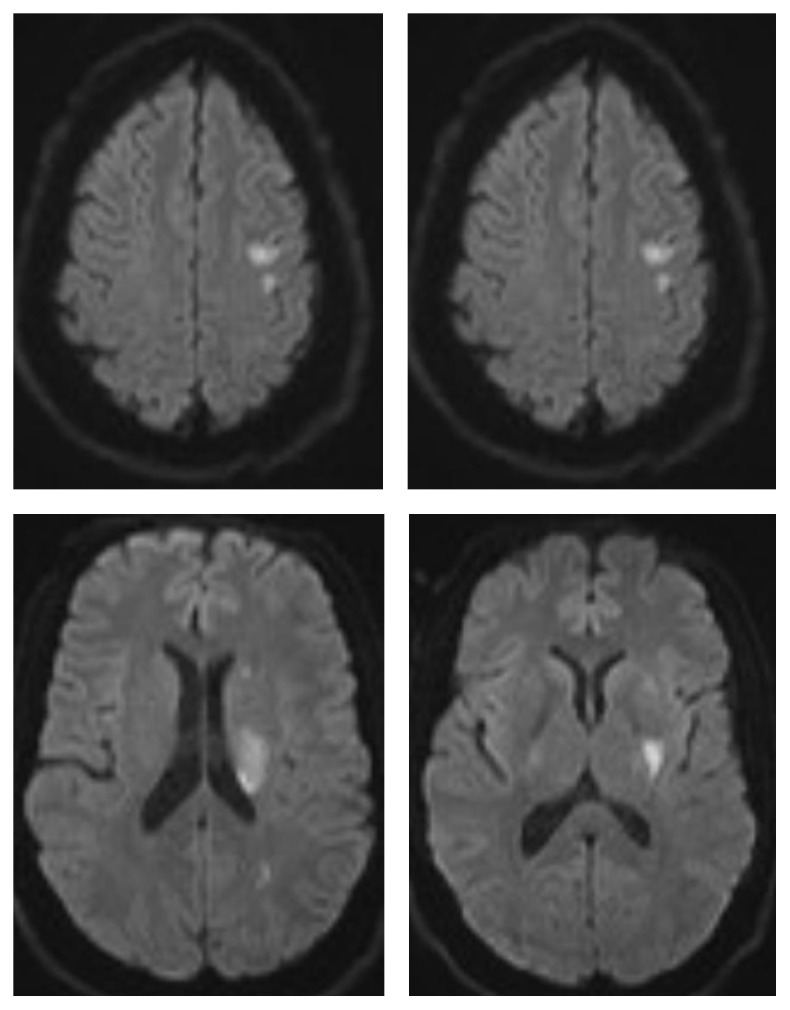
MRI brain DWI sequence demonstrating restriction diffusion in left middle cerebral artery distribution.
